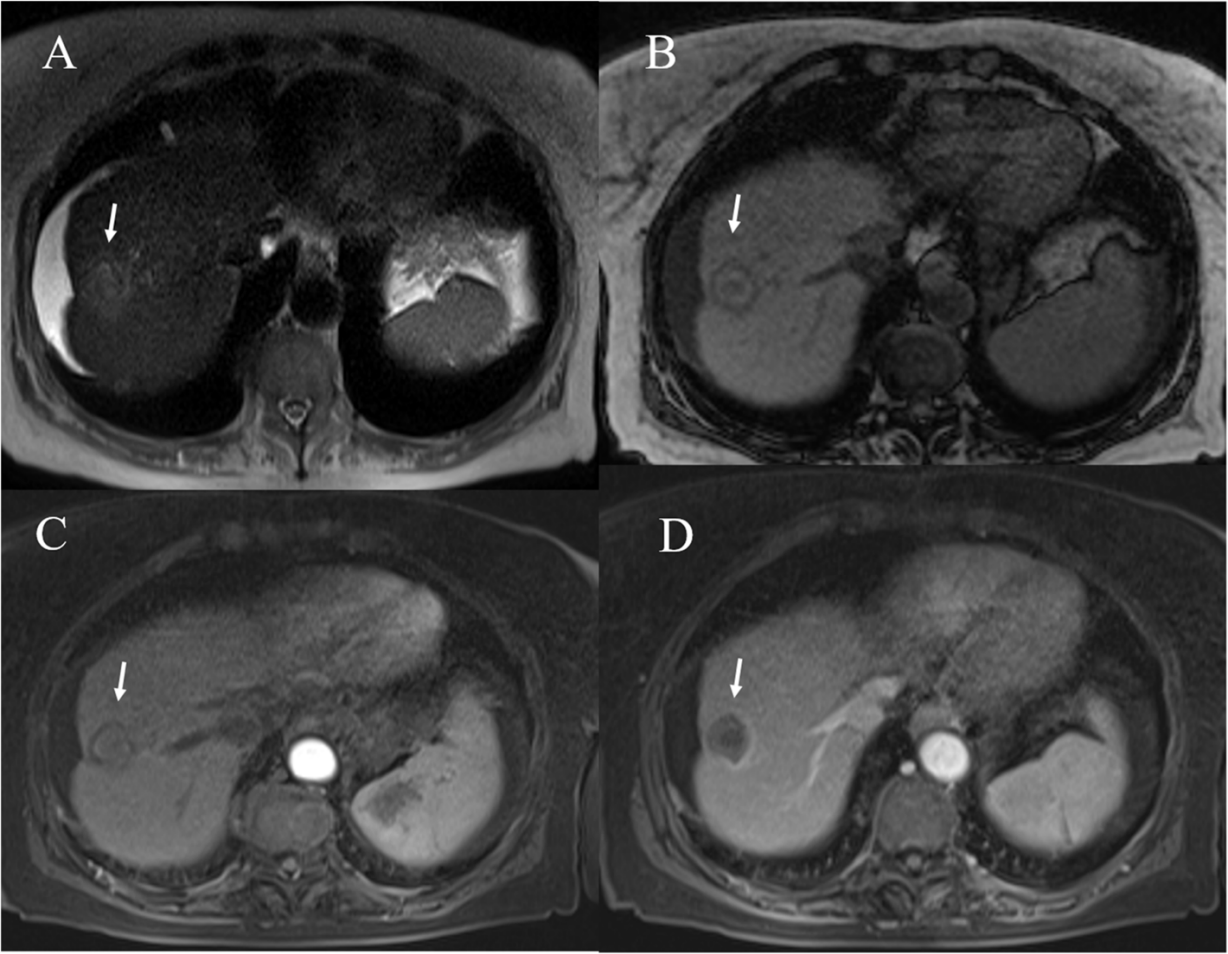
MRI assessment of HCC on II hepatic segment. The lesion show (arrow) hyperintense signal in T2-W sequence (A), hyperehancement during arterial phase (B), wash out appearance (C) during portal phase and nodule in nodule appearance in hepatobiliary phase (D)
